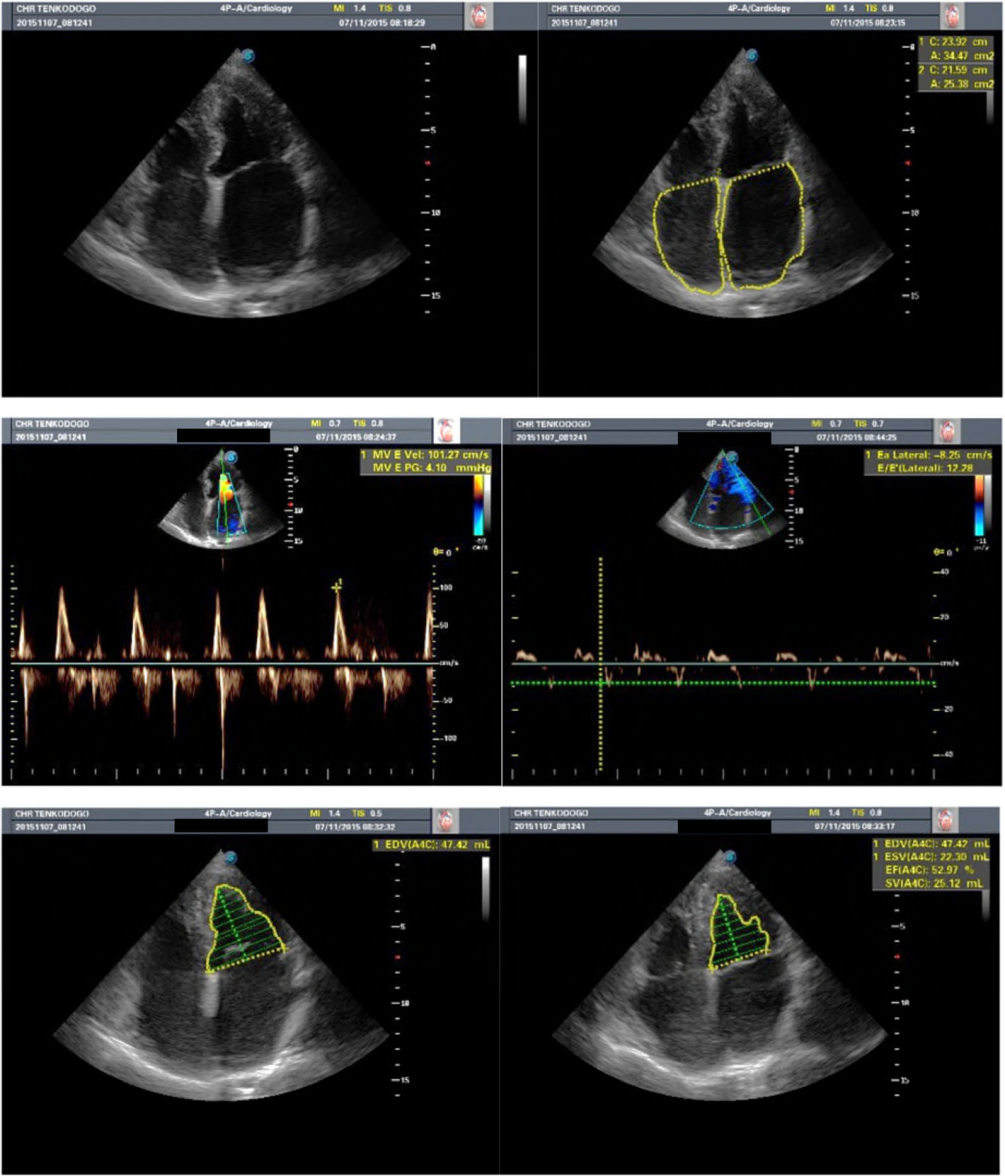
Echocardiogram revealing restrictive cardiomyopathy in a 78-year-old woman with atrial fibrillation. Note the reduction of both ventricles’ volumes, normal left ventricle (LV) wall thickness and massive bi-atrial enlargement in apical four-chamber views. The LV ejection fraction was slightly reduced with evidence of diastolic dysfunction
